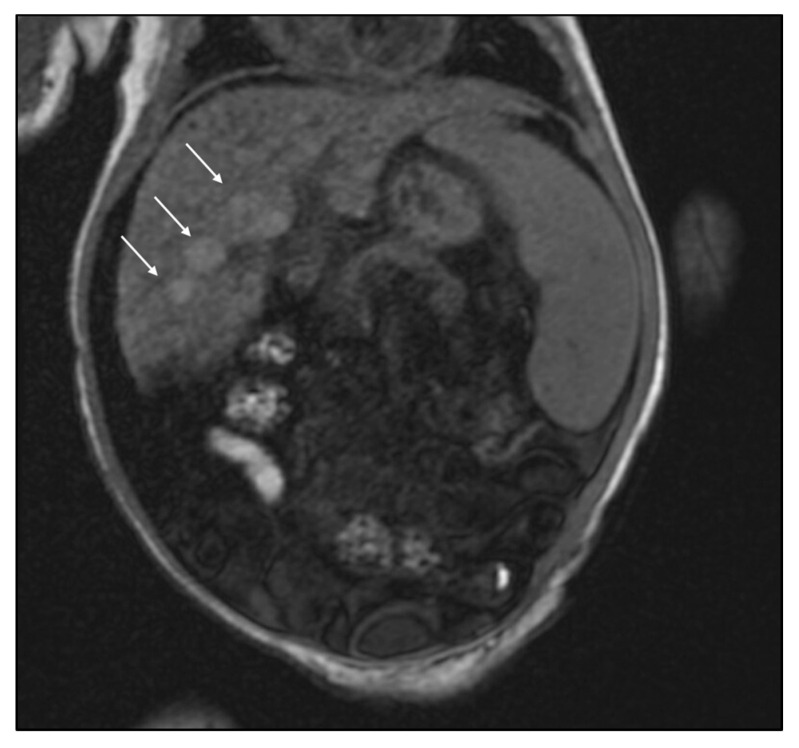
Non-contrast, T1-weighted abdominal MRI from DOL 68 showing the patient’s liver enlargement and irregular surface consistent with cirrhosis and multiple regenerative nodules (white arrows). The spleen is also enlarged, and there is abdominal distention secondary to ascites.
